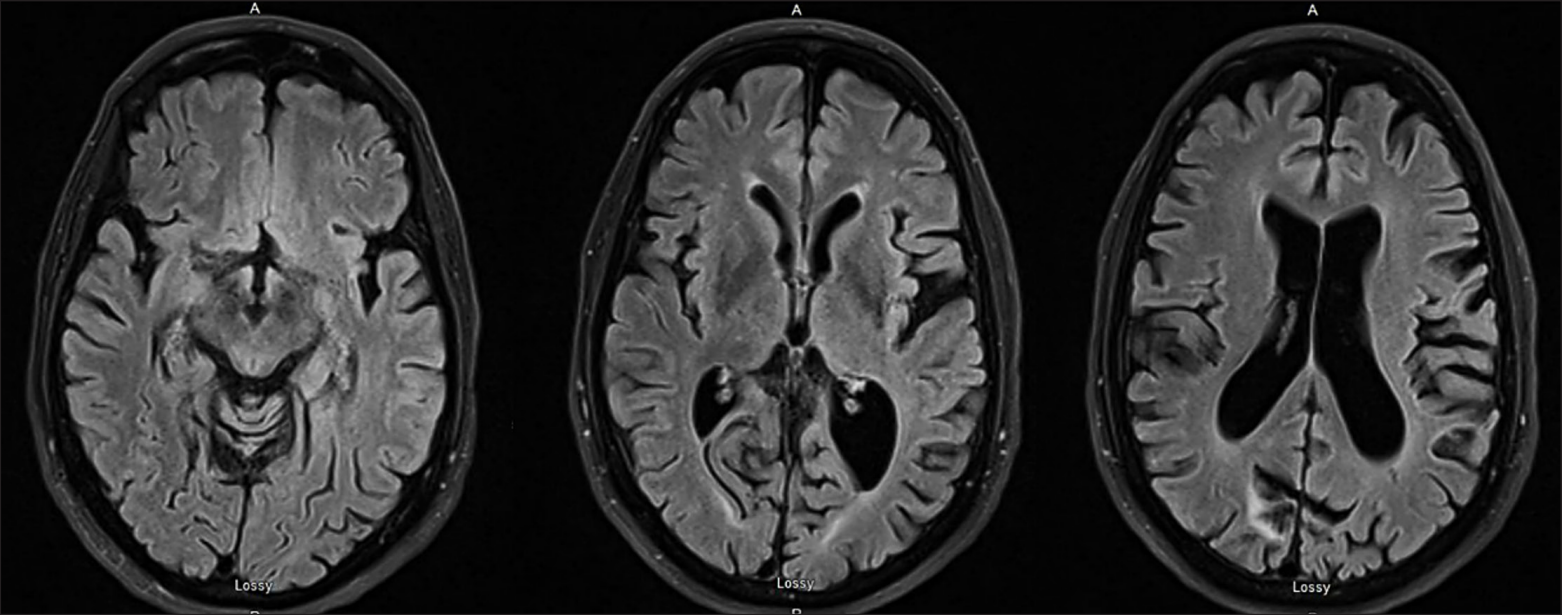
MRI brain. Preoperative MRI revealing late sequela of demyelination with brain atrophy and pathy areas of secondary axonal loss throughout the brain and concavity of the apex of the midbrain.
